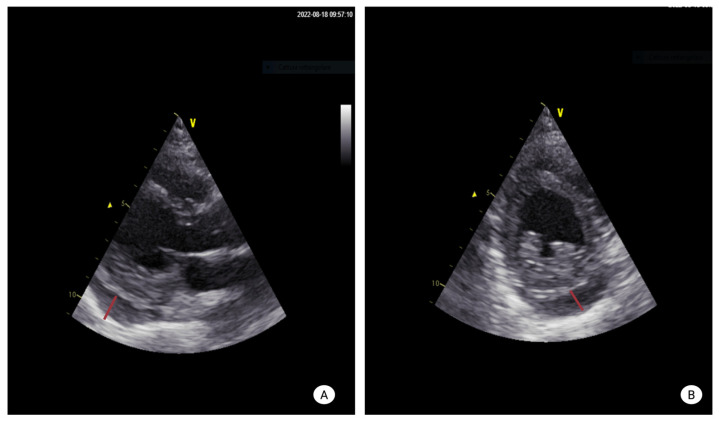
Imaging features of severe pericardial effusion in a 40-years- old SLE. (A) Transthoracic echocardiography, parasternal long-axis view, showing a large pericardial effusion predominantly along the posterolateral wall. (B) Parasternal short-axis view confirming the presence of a circumferential pericardial effusion, more evident along the lateral wall.
